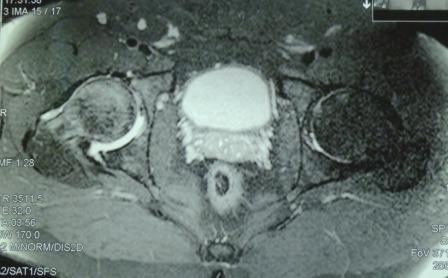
Preoperative MRI, showing the aseptic femoral head necrosis
